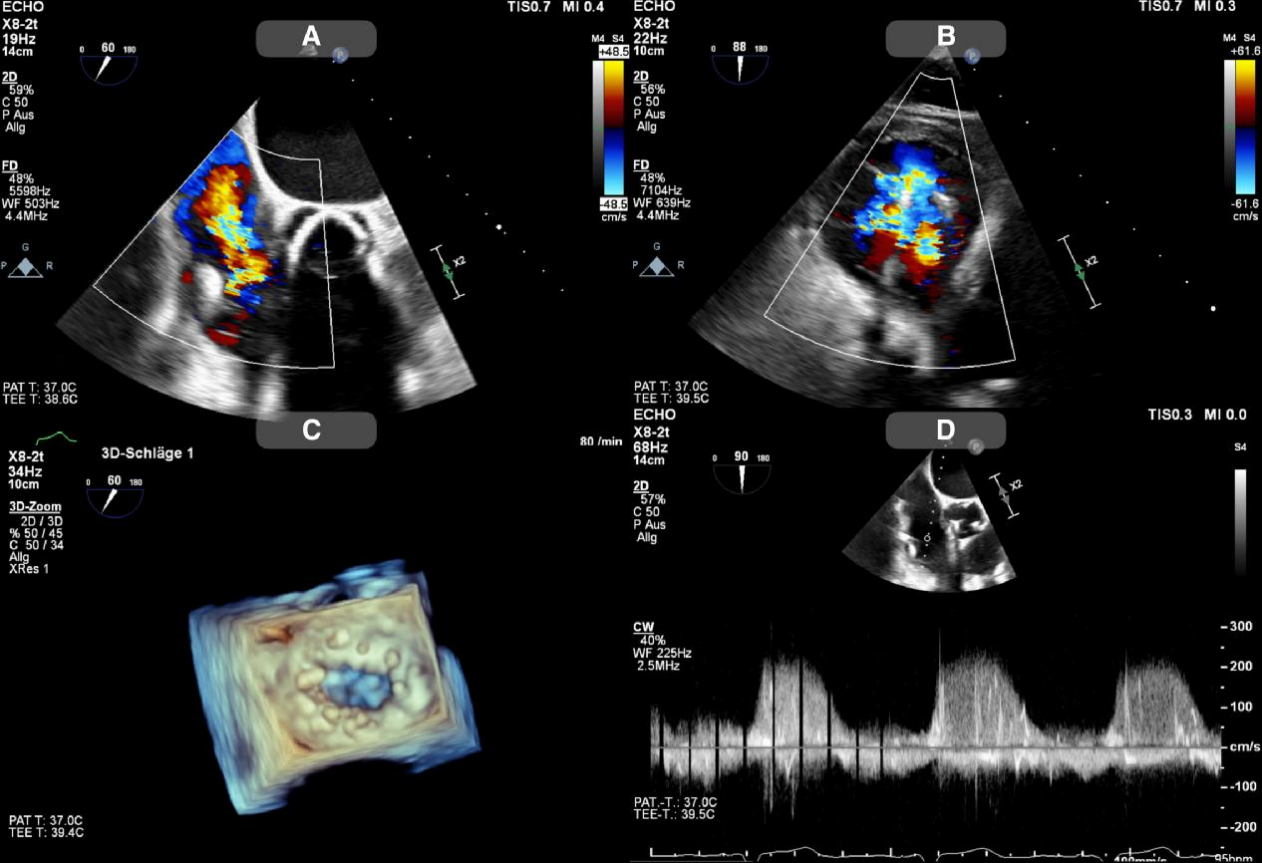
Pre-procedural echocardiographic assessment shows pre-procedural echocardiographic images of the tricuspid regurgitation. (*A* and *B*) Colour Doppler images of the torrential tricuspid valve insufficiency. (*C*) 3D reconstruction of the large central coaptation gap. (*D*) Tricuspid regurgitation velocity within the normal range, thereby indicating no elevated pulmonary pressure.
